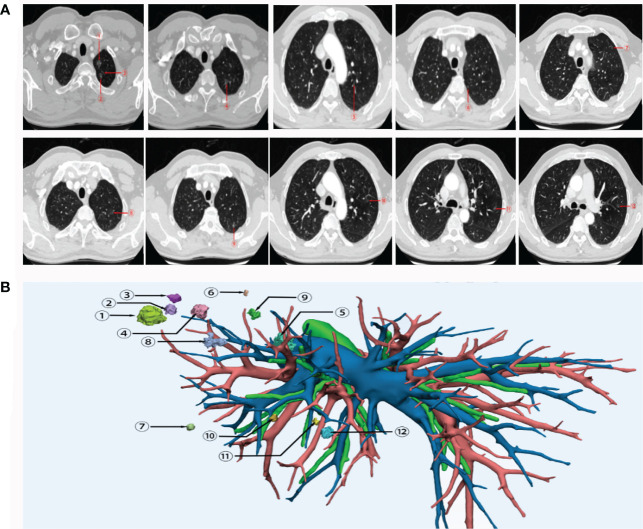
CT images (A) and 3D reconstruction (B) of this patient with early-stage MPLC. Nodule 1, nodule 3, nodule 5, nodule 6 and nodule 9 were identified as MIA. Nodule 4, nodule 12, and nodule 11 were identified as AIS. No cancer cells were found in nodule 2, nodule 7, nodule 8, and nodule 10. MPLC, multiple primary lung cancer; AIS, adenocarcinoma in situ; MIA, minimally invasive adenocarcinoma.
